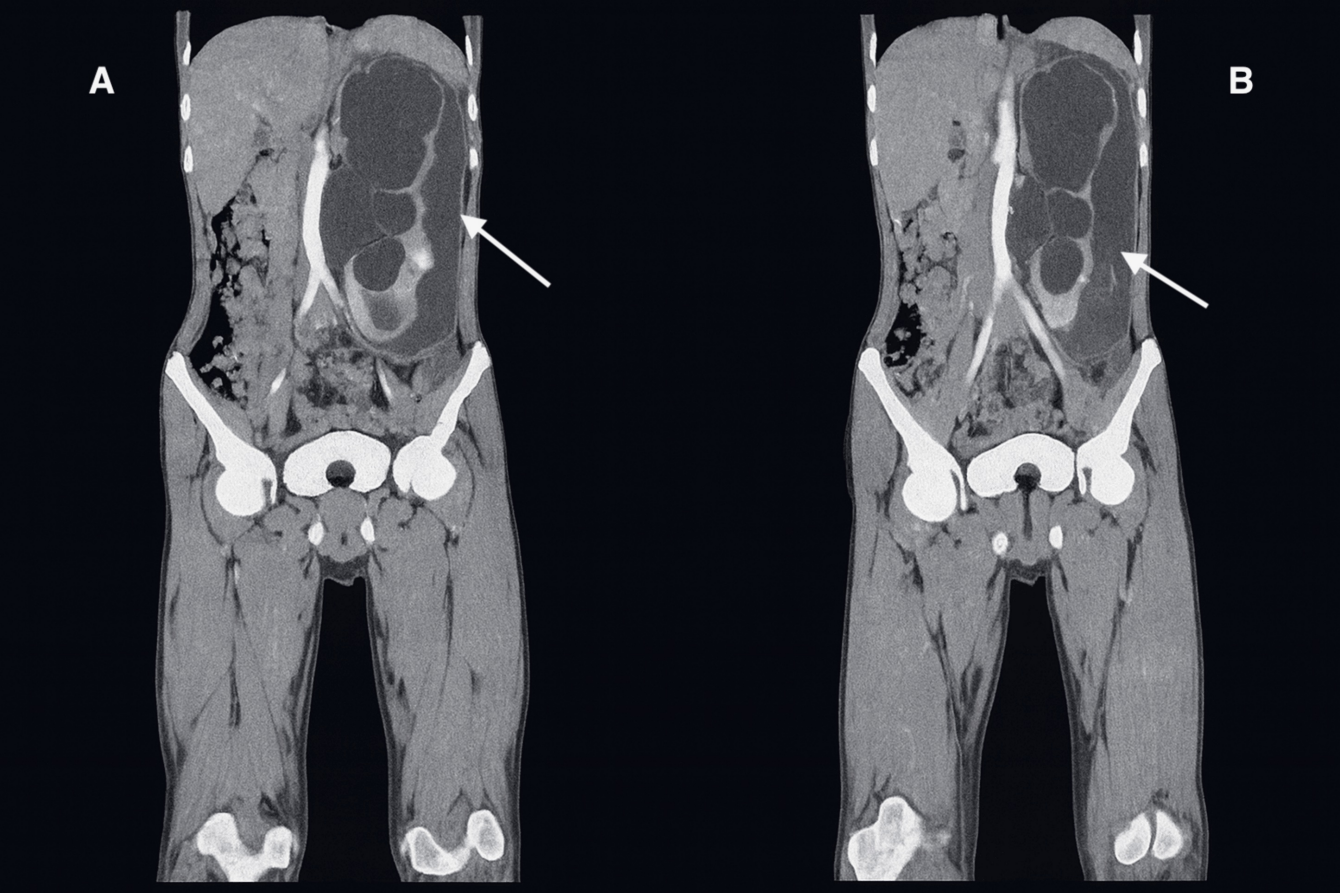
CT urogram coronal view Coronal CT images show the spontaneous rupture of a hydronephrotic kidney. A: Markedly dilated pelvicalyceal system with thinning of renal parenchyma; B: Perinephric fluid collection consistent with urinary extravasation. These findings are consistent with spontaneous pelvicalyceal rupture secondary to high-pressure obstruction.
